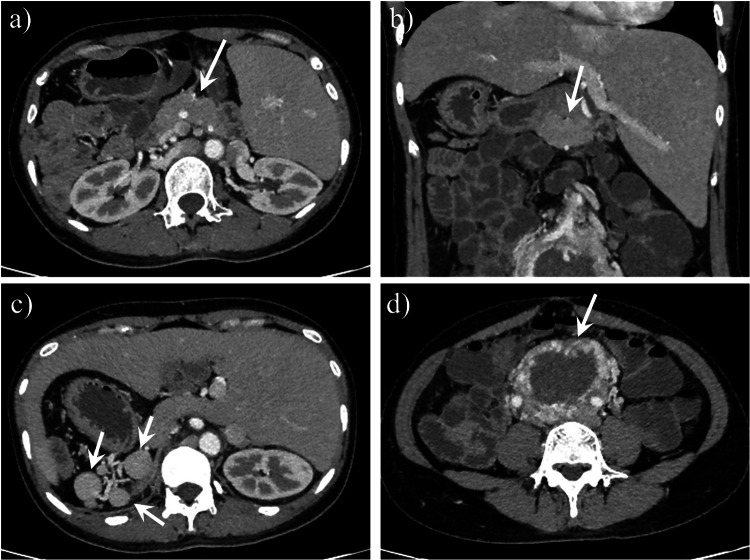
A 35-year-old woman had dorsal pancreatic agenesis, polysplenia syndrome, total situs inversus viscerum and retroperitoneal paraganglioma. Axial (a) and coronal (b) contrast-enhanced computed tomography (CT) scans showed the pancreatic head and neck (arrow) and the absence of the pancreatic body-tail portion. Axial contrast-enhanced CT scan (c) showed multiple accessory spleens in the right upper quadrant (arrow), and most of the liver was located in the left upper abdomen. Axial contrast-enhanced CT scan (d) showed a markedly inhomogeneous enhanced retroperitoneal mass (arrow), which was pathologically confirmed as a paraganglioma
